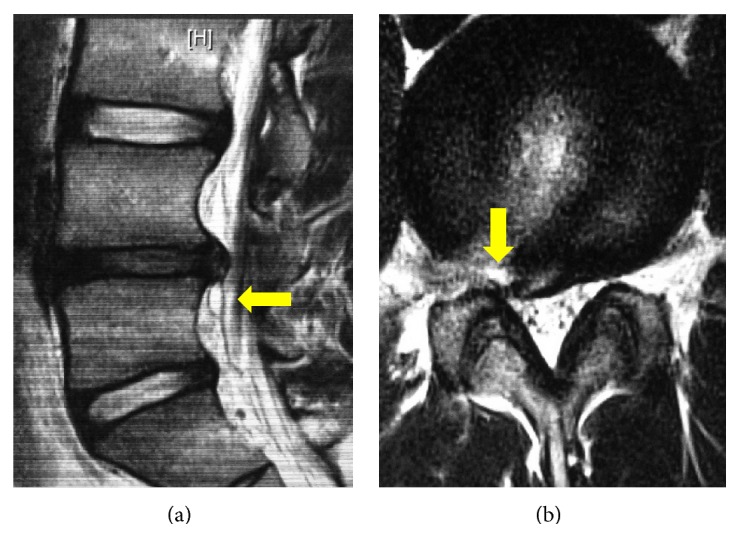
Postoperative magnetic resonance imaging at the 1-month follow-up: (a) sagittal T2-weighted and (b) axial T2-weighted images demonstrating the irregular posterior margin of the L4-L5 disc and decrease in size of the cyst (arrows).
